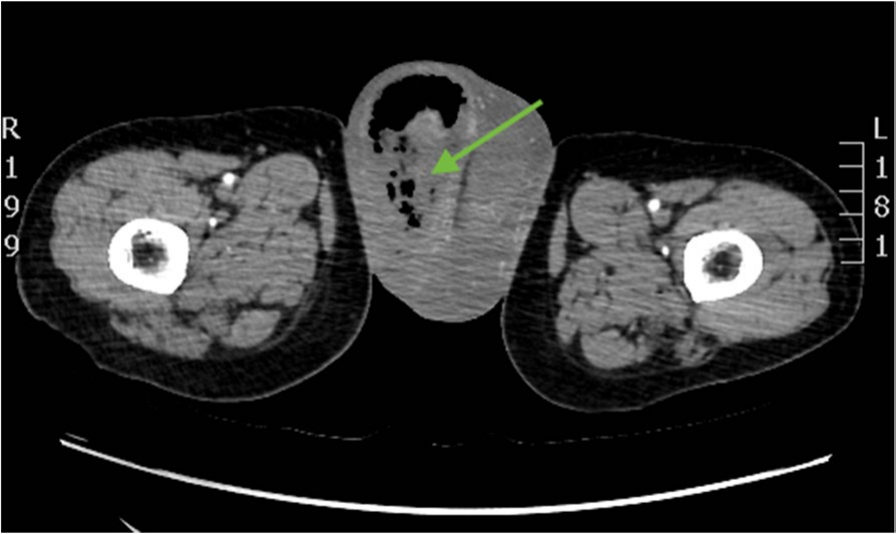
Title. Pre-operative CT-scan. CT-scan revealed air-gas content (green arrow) in the context of the soft and peripheral tissues at the level of the right scrotal lodge. A marked fluid-edematous thickening of the tunics and scrotal walls were present bilaterally but more evident on the right side of the scrotum
